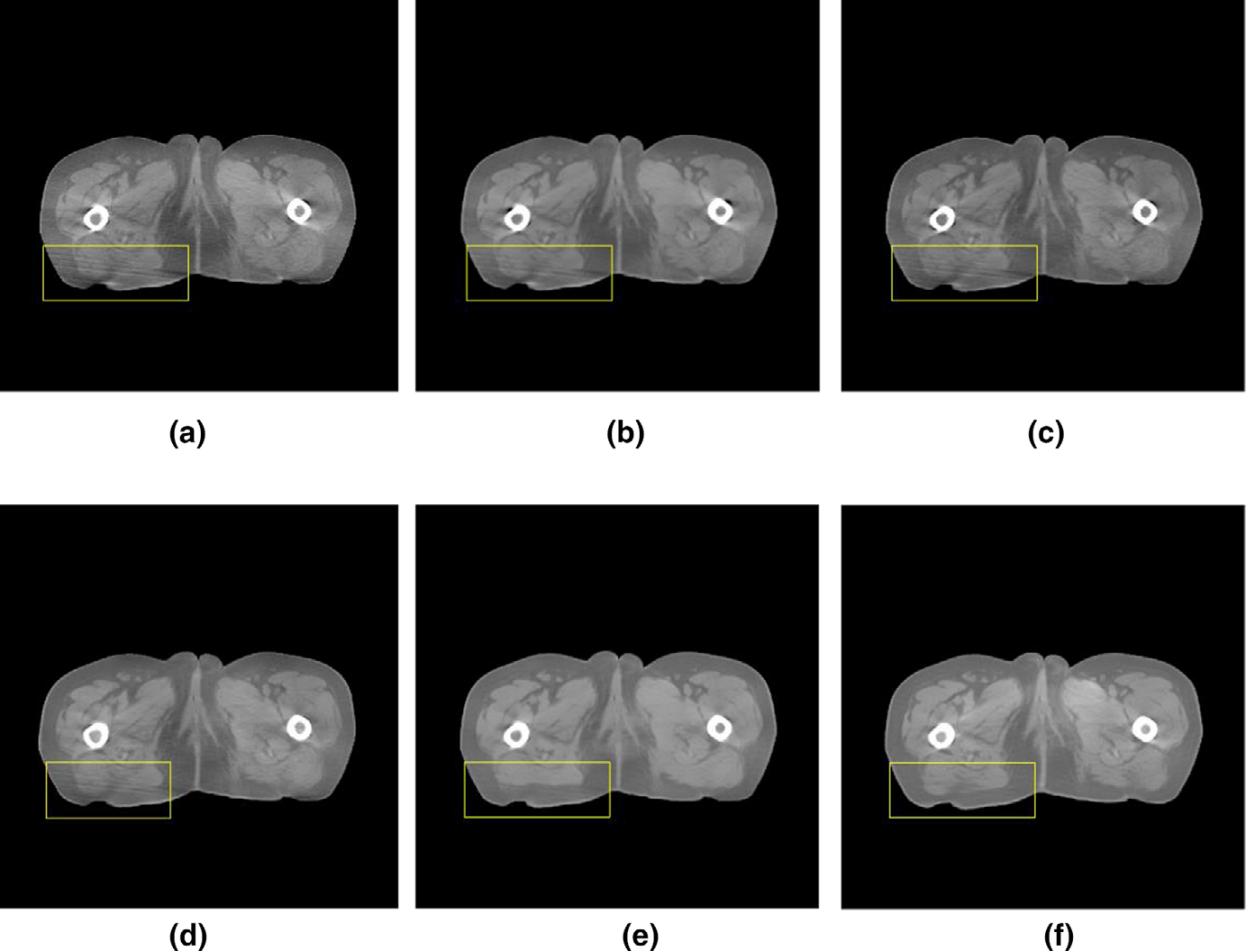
Artifact removal results obtained in optimization process. (a) CBCT, (b) Step 1, (c) Step 2, (d) Step 3, (e) Step 4, and (f) Step 5. Display window [−502, 528].
